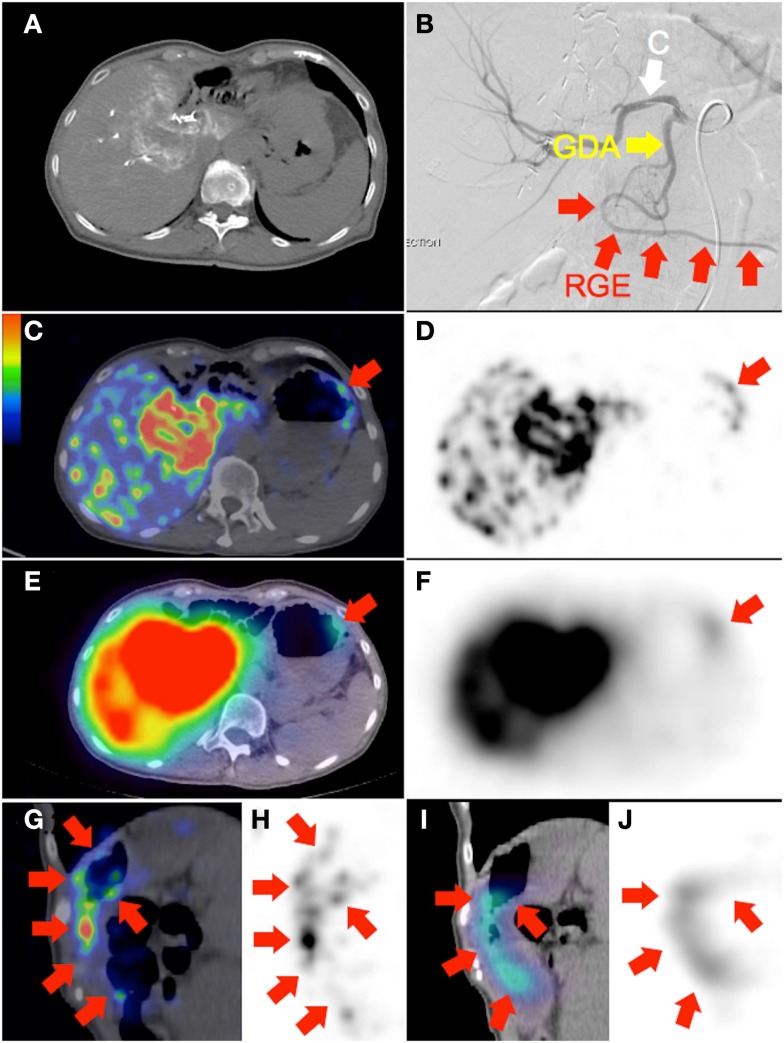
(A) Recurrent cholangiocarcinoma at the surgical margin of a previous left hemi-hepatectomy, seen on catheter-directed CT hepatic angiogram of the right hepatic artery. RE was performed with the catheter tip in the right hepatic artery, beyond the origin of the gastroduodenal artery. Prophylactic coil embolization of the gastroduodenal artery was not performed at the discretion of the interventional radiologist. (B) Post-RE digital subtraction angiogram with the catheter tip (“C”) position unchanged demonstrates significant vascular stasis and reflux of contrast in the common hepatic, gastroduodenal (“GDA”), and right gastroepiploic (“RGE”) arteries. (C,D,G,H)
